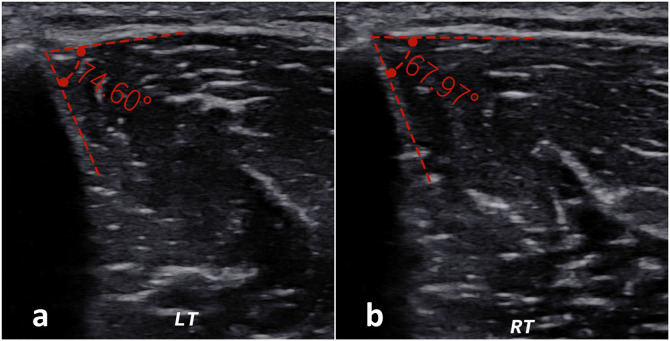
Ultrasound measurement of the tibia-fascia angle (TFA). Representative images are from a 40-year-old male with a Schatzker type II tibial plateau fracture sustained in an electric bicycle accident (intracompartmental pressure: 28 mmHg). (a) Affected side (left) showing a TFA of 74.60°; (b) Contralateral (unaffected) side showing a TFA of 67.97°. The TFA is defined as the angle subtended by the anterolateral tibial cortex and the tangent to the anterior compartment fascia, with the vertex at the fascial attachment.
